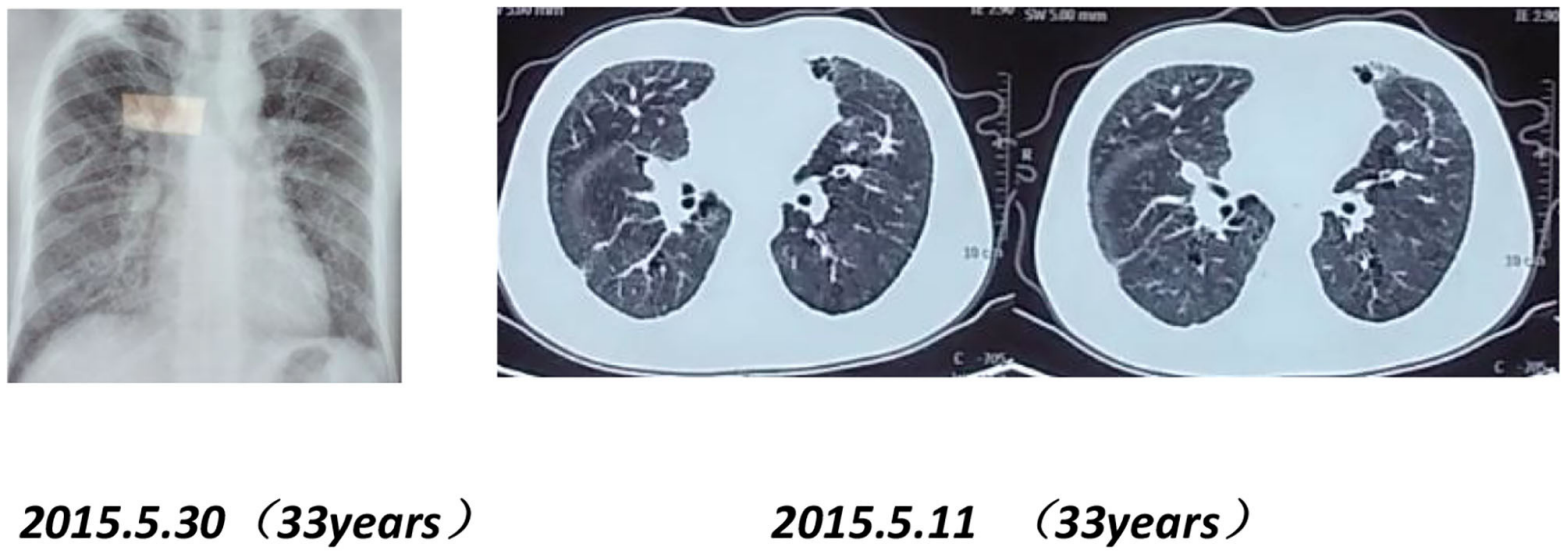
Chest X-rays and CT scans of the proband's father (33 years old) show interstitial pulmonary inflammation.
